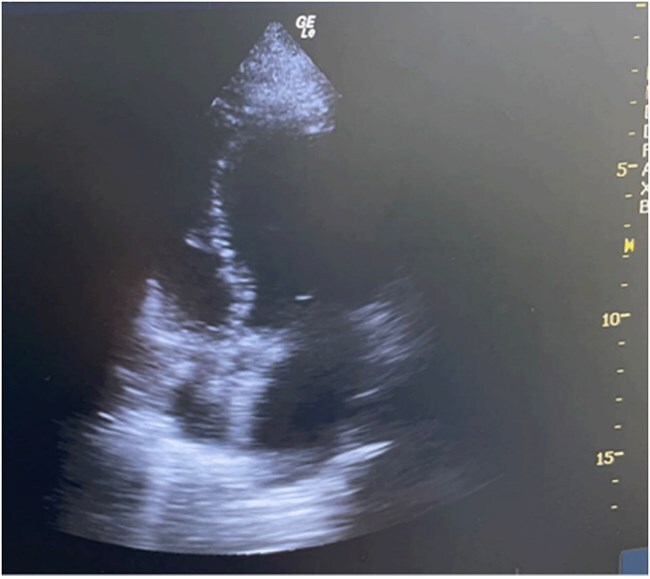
Bedside echocardiography revealed left ventricular dilation, diffuse hypokinesia, and a reduced ejection fraction of 30%.
